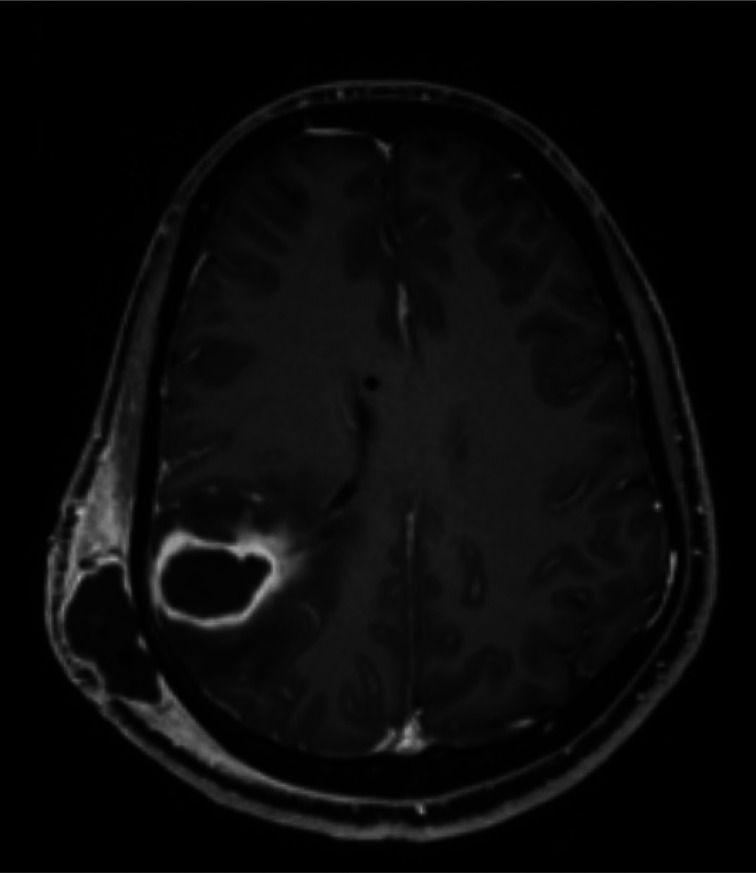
Contrast MRI showing a ring-enhanced lesion within the previous VPS pathway and connected to the subcutaneous tissue
